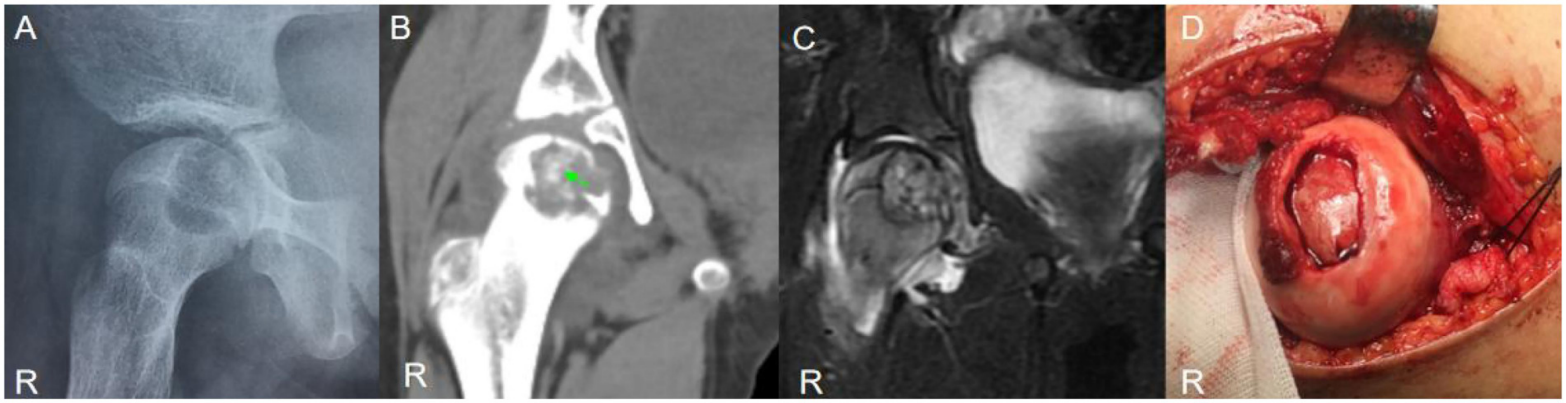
Case 4: (A) an isolated eccentric osteolytic lesion in the right femoral head. (B) CT (soft tissue window): Irregular calcified tissue (green arrow). (C) MRI (T2WI-STIR): high signal in the femoral head on the tumor side, with irregular signals within the tumor. (D) After curetting the tumor tissue under direct visualization, autologous iliac bone was harvested for grafting to support the cavity and repair the damaged articular surface.
